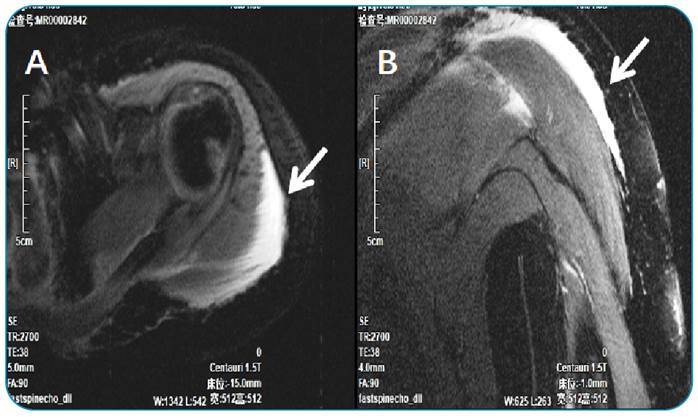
(A, B): MRI scans reveal swelling in the deltoid, trapezius, and supraspinatus muscles and subcutaneous fat layer (arrow).
